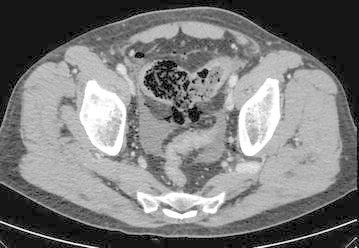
Abdominal CT-scan showing endoperitoneal free air, some thickened ileal loops and pelvic free fluids with a large pelvic collection..
